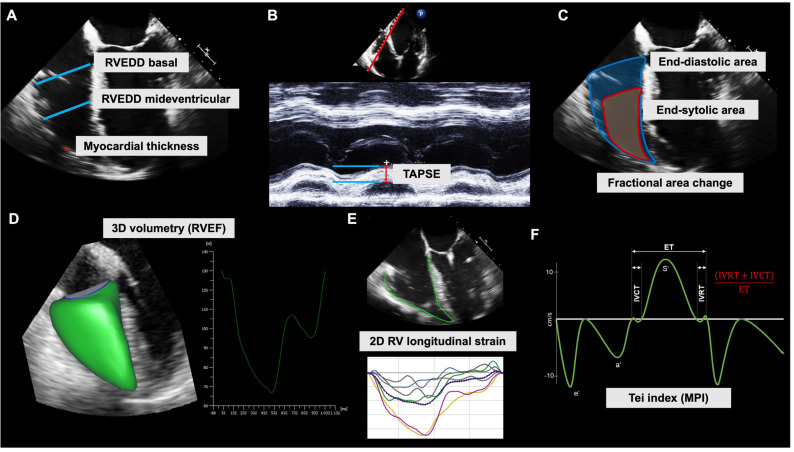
Analysis of right ventricular function. (A) Measurement of the enddiastolic diameter of the right ventricular (RVEDD) and the myocardial thickness of the free wall in the four-chamber mid-esophageal view provides a rapid analysis of the dimensions of the right ventricular. (B) Tricuspid annular plane systolic excursion (TAPSE, mm) using M-mode in apical four-chamber view reflects longitudinal right ventricular systolic function. (C) The relative change between the end-diastolic and end-systolic endocardial right ventricular area is called the fractional area change (FAC). FAC can be obtained in four-chamber transthoracic or transesophageal views and is a measure of systolic function. (D) Three-dimensional acquisition of the whole right ventricle and speckle-tracking-based analysis yields RV volumes, stroke volume, and ejection fraction (RVEF), avoiding geometrical assumptions and confounding 2D artifacts. (E) Strain analysis using speckle-tracking allows for the quantification of myocardial deformation, e.g., 2D RV longitudinal strain of the septal and free wall. (F) The Tei index (or myocardial performance index, MPI) is derived from isovolumetric relaxation time (IVRT), isovolumetric contraction time (IVCT), and ejection time (ET). This measure of global right ventricular function is usually calculated automatically after the manual definition of IVRT, IVCT, and ET in the tissue doppler profile of the basal right ventricular free wall.
